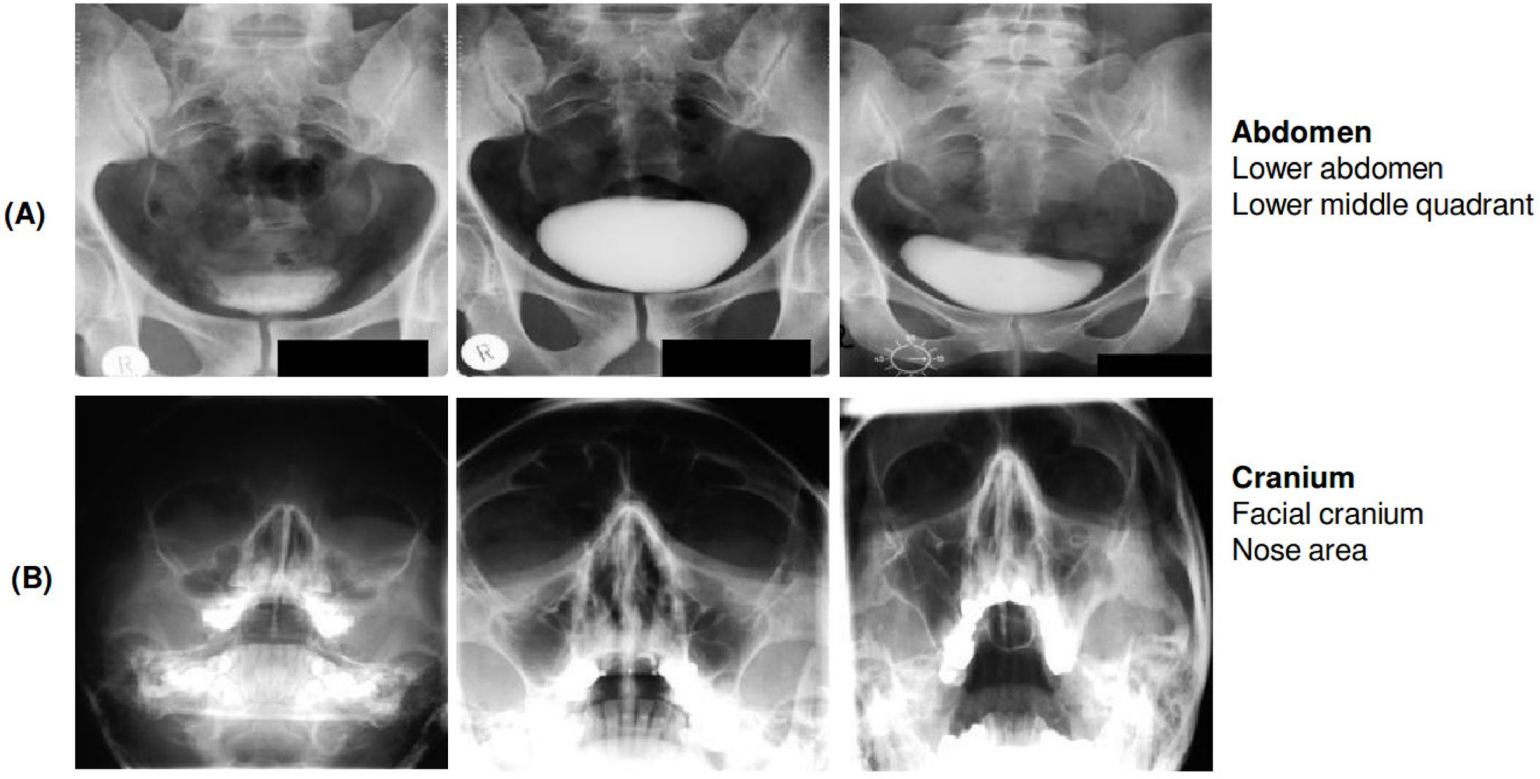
Examples of radiographs annotated with two classes from the A-scheme. (A) shows three images each belonging to class ‘732’ representing ‘Abdomen; Lower abdomen; Lower middle quadrant’ and (B) displays three images belonging to ‘213’ representing ‘Cranium; Facial cranium; Nose area’. All radiographs were randomly chosen from the ImageCLEF 2009 Medical Annotation Task Training Set. Republished from [21] under a CC BY license, with permission from [RWTH Aachen], original copyright [2009].
